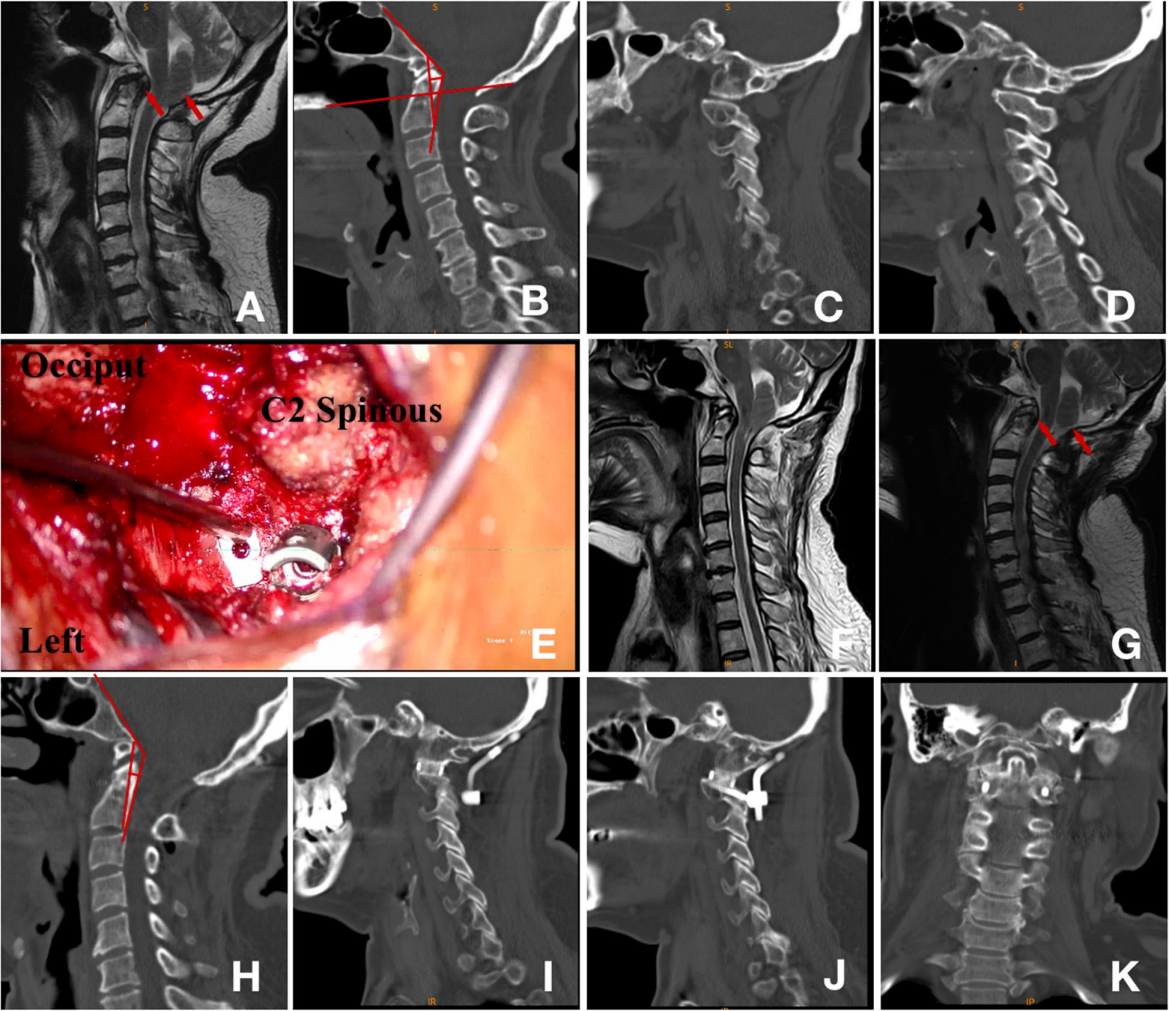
A 66-year-old woman presented with sensory disturbance and unsteady walking for more than 4 years. After the operation, the patient’s unsteady walking was significantly relieved, and the Japanese Orthopedic Association (JOA) score increased from 11 to 15. A: Preoperative sagittal magnetic resonance imaging (MRI) showed tonsillar hernia, large soft tissue volume behind the odontoid process, and ventral compression of the brain stem. B: Preoperative sagittal computed tomography (CT) showed basilar invagination (BI), occipitalization of C1, clivus-canal angle (CCA) = 130.6°, pB-C2 = 6.5 mm, and no deformity of the bilateral lateral atlantoaxial joint C, D. E: During the operation, the cage was placed between the joints and then screws were placed. F: Sagittal MRI on the third day after operation showed that the compression on the ventral side of the brain stem was reduced compared with that before operation, and the descending degree of the tonsillar hernia had no significant change compared with that before operation. G: Three months after operation, MRI showed that the descending degree of tonsillar hernia was significantly reduced, the volume of soft tissue behind the odontoid process was smaller than that before operation, and the ventral compression of brain stem was relieved. H: Six months after operation, CT scan showed increased CCA, CCA = 137.5°, pB-C2 = 4.4 mm, and bone fusion can be seen between the joints of the bilateral lateral atlantoaxial joint I, J, K
